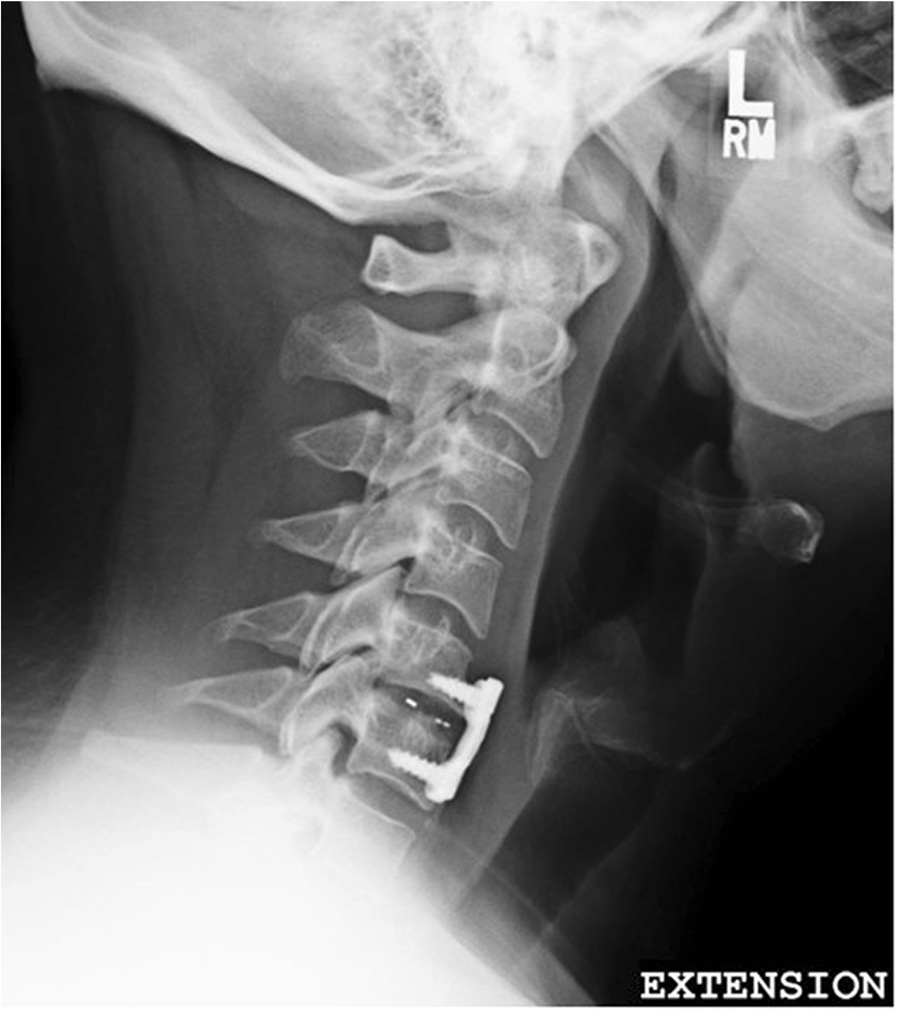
Post-operative X-ray image. Lateral projection showing anterior cervical fusion at the C5 to C6 level.
